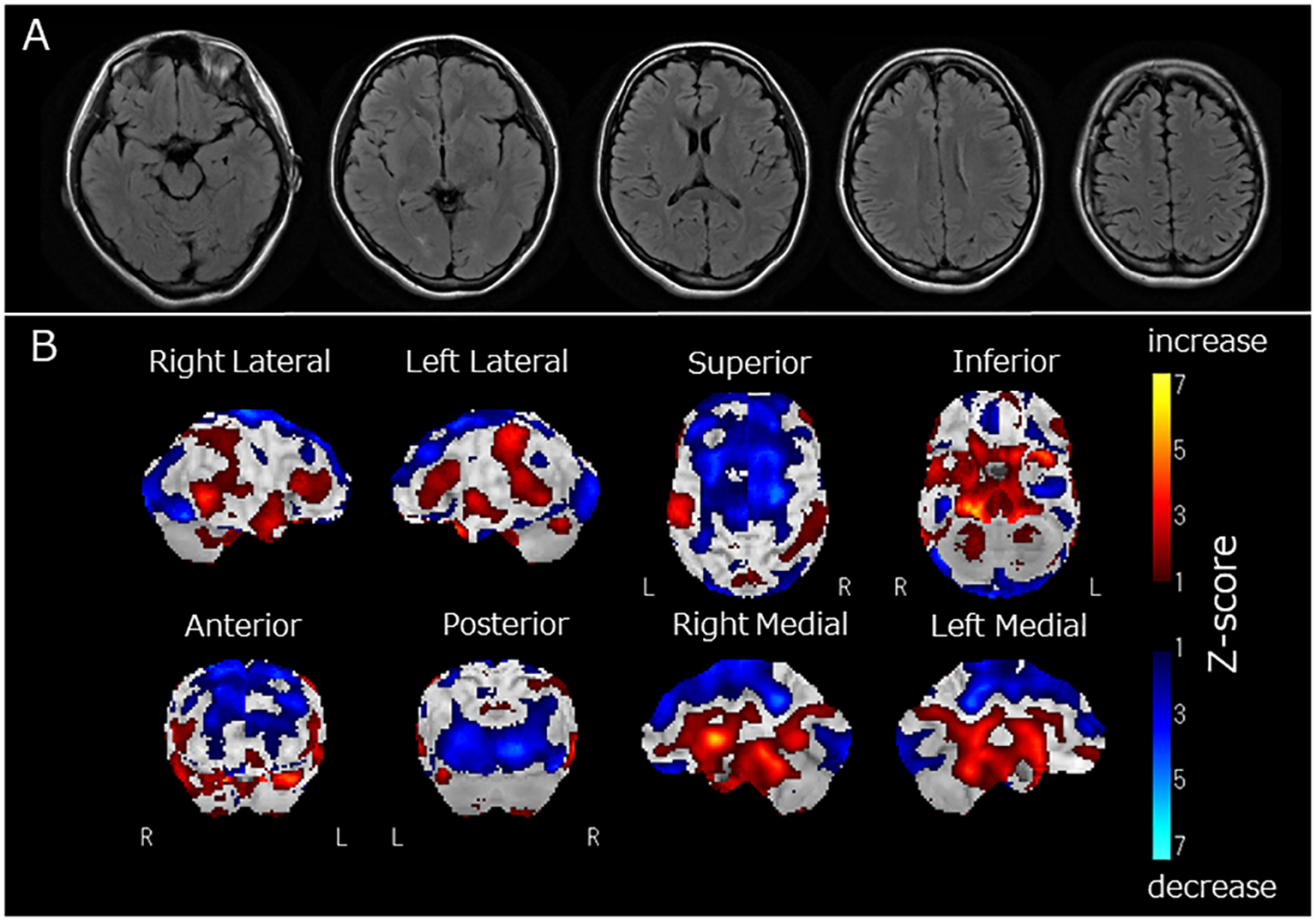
(A) Fluid Attenuated Inversion Recovery magnetic resonance imaging of the brain, (B) regional cerebral blood flow (rCBF) using single-photon emission computed tomography (SPECT) with ^123^I-iodoamphetamine. Fluid-attenuated inversion recovery magnetic resonance imaging revealed no apparent brain atrophy or high-intensity areas (A). SPECT using a ^123^I-iodoamphetamine was performed, and SPECT data were analyzed by 3D stereotactic surface projections (3D-SSP) included in “medi+FALCON version 1.4” (Nihon Medi-Physics Co. Ltd., Tokyo, Japan). Z-score maps of regional cerebral blood flow (rCBF) were created based using 3D-SSP. The SPECT images showed decreased rCBF in the occipital and frontal lobes (B). In contrast, rCBF increased in the basal ganglia and pons (B).
